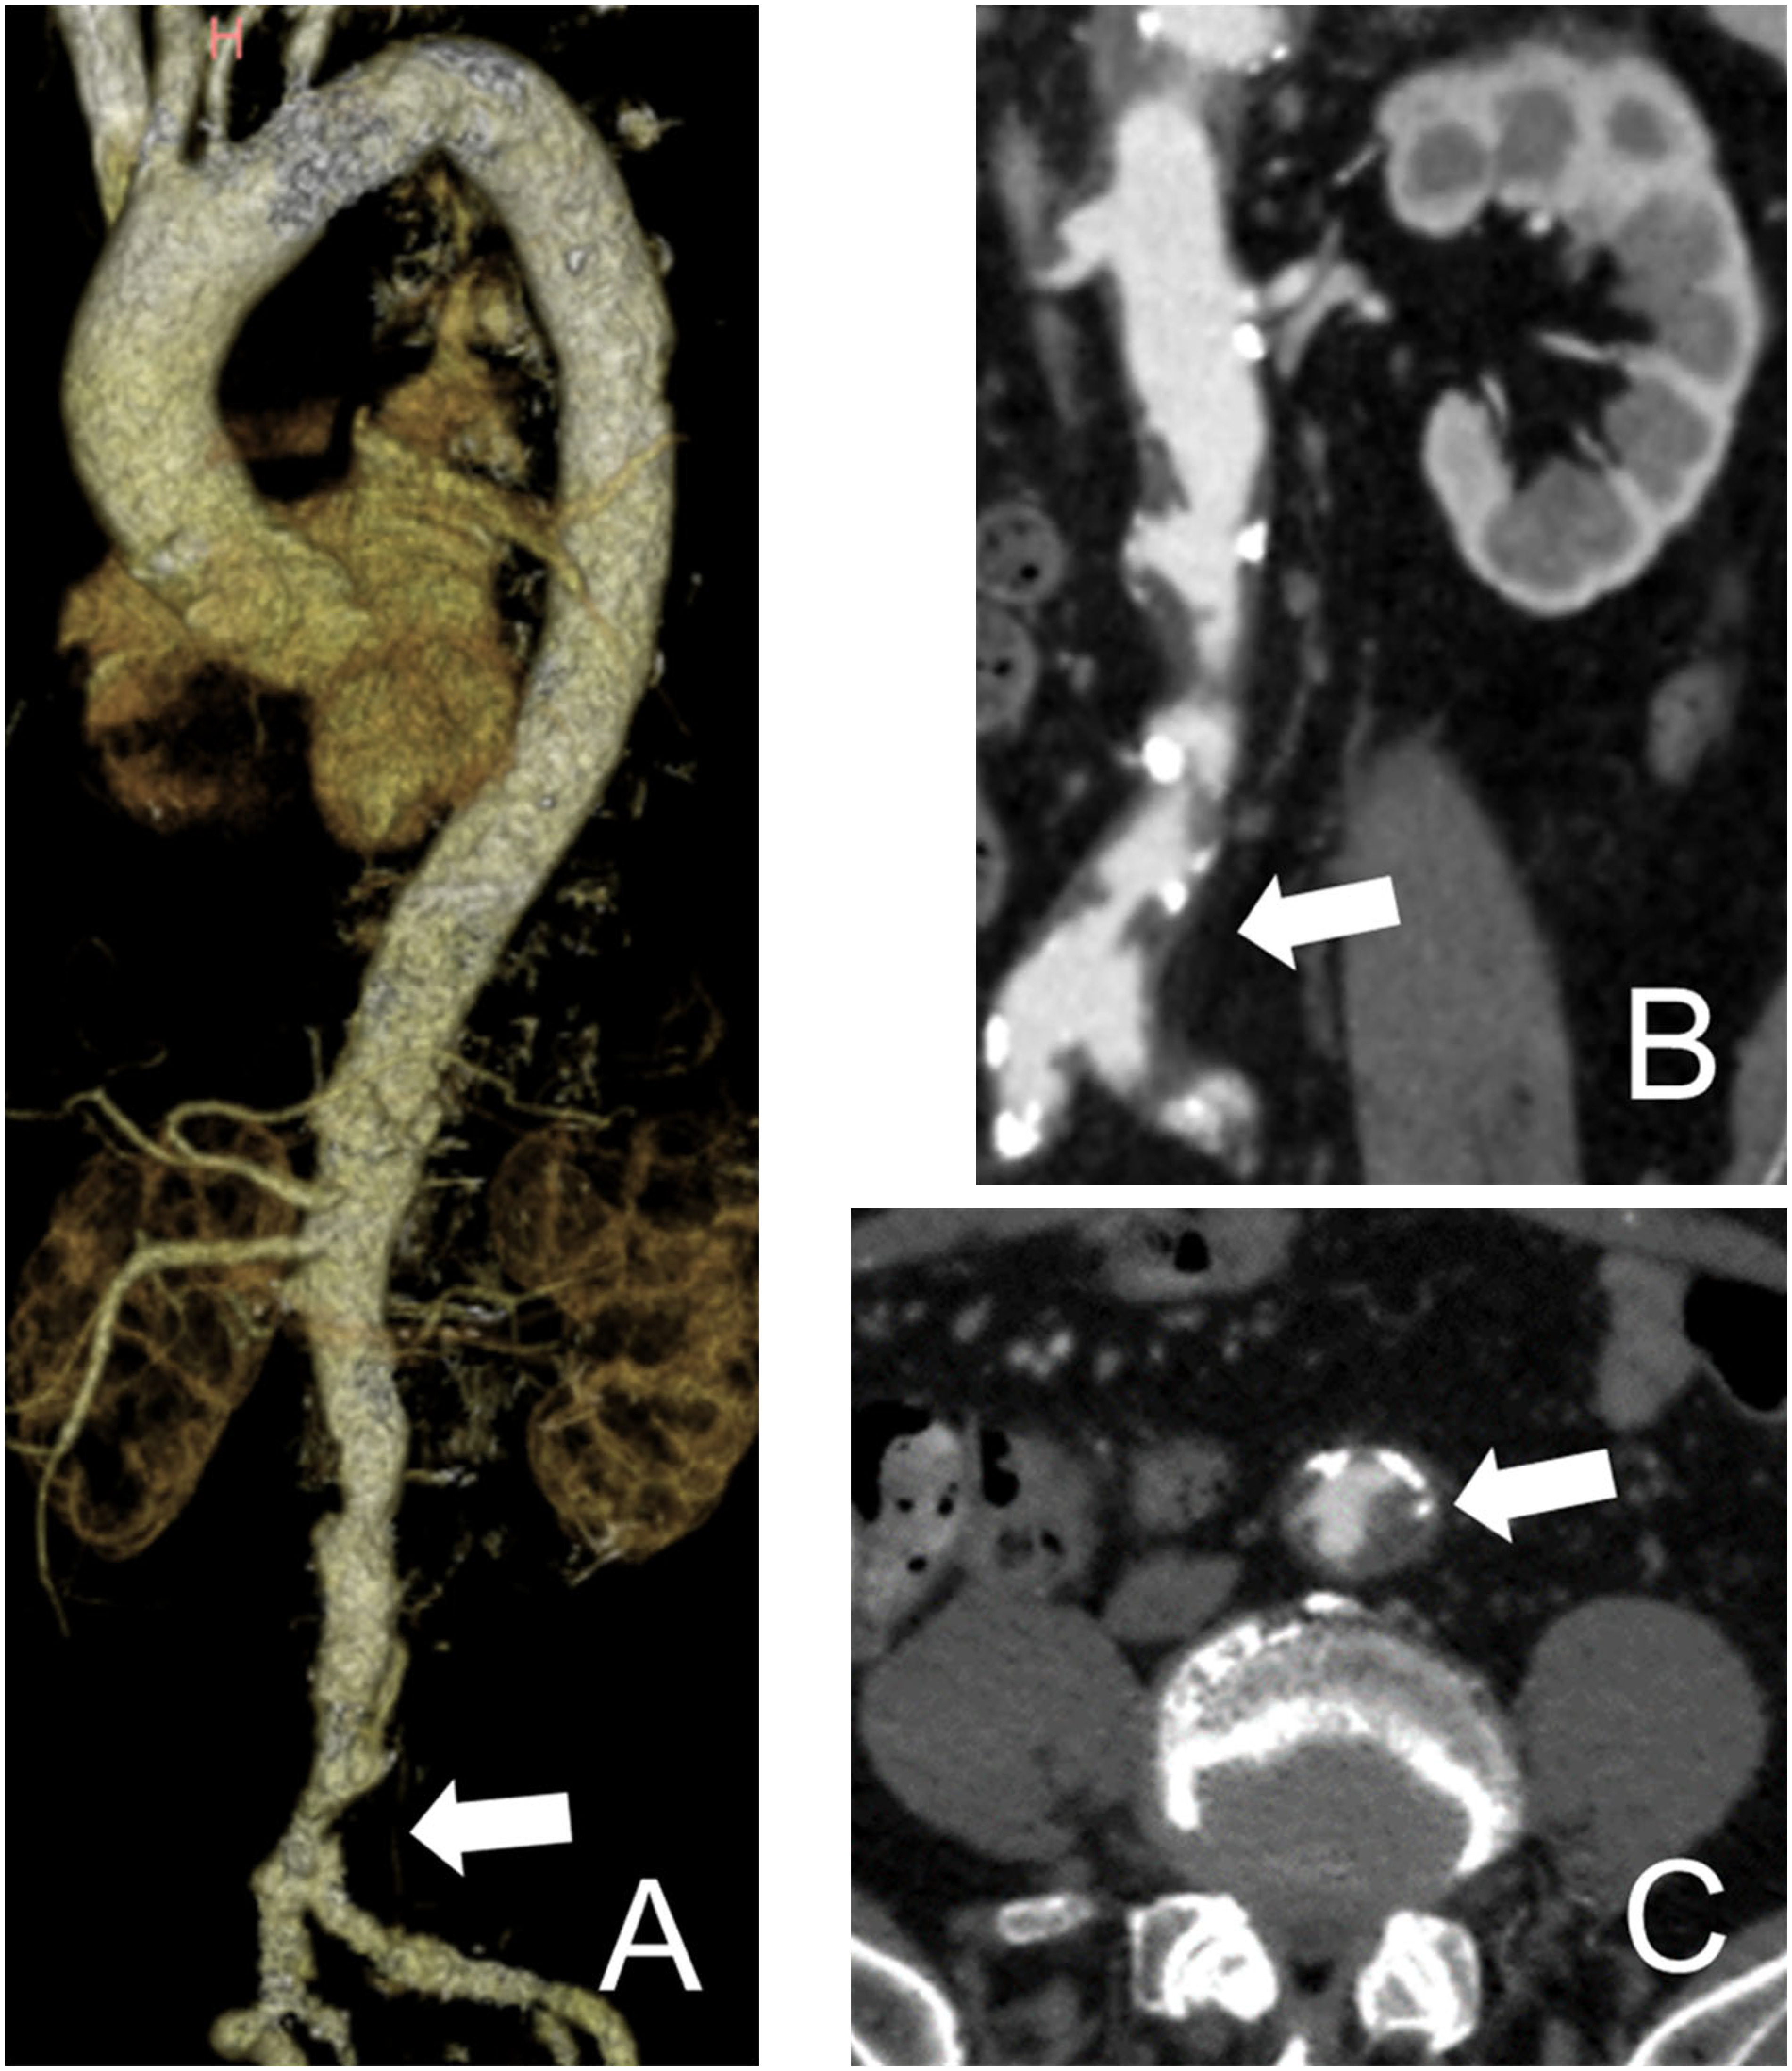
Fig. 1 (A) Three-dimensional computed tomography (CT) image. (B) Multiplanar reconstruction image. (C) Cross-sectional image of a preoperative enhanced CT scan. The atherothrombosis is floating in the abdominal aorta.
